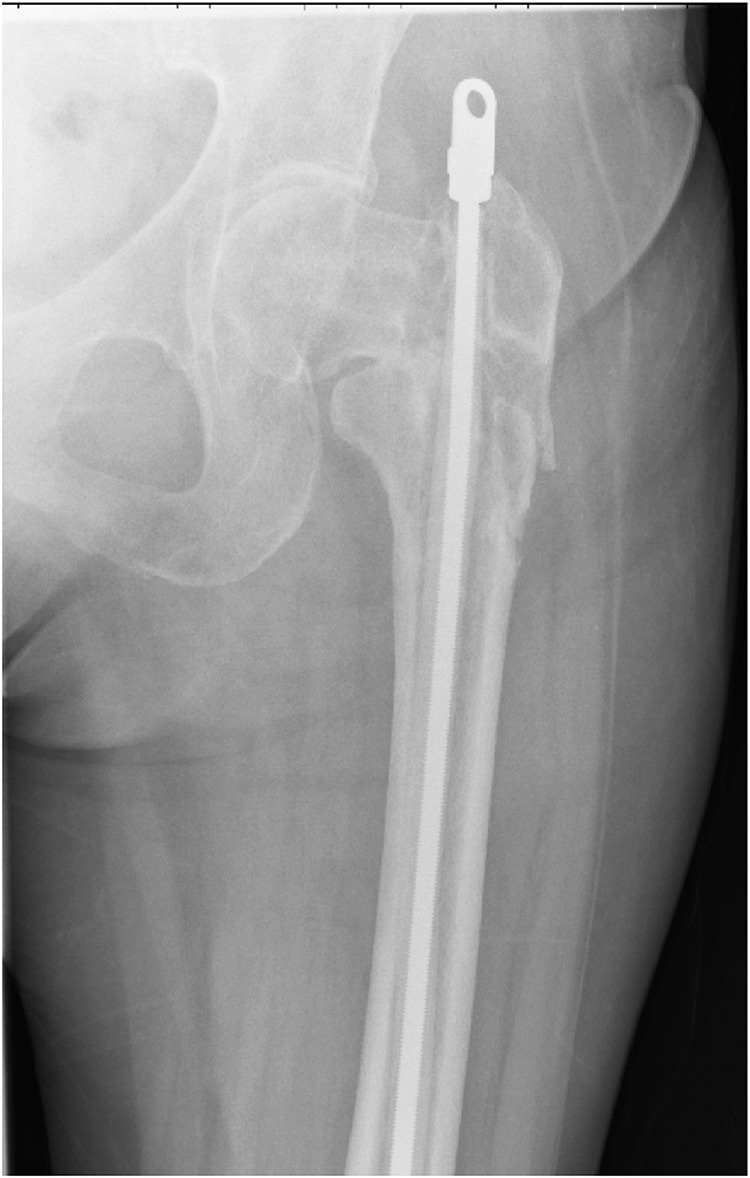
X-ray showing Ilizarov rod with cement used as temporary spacer following removal of infected left femoral intramedullary nail
